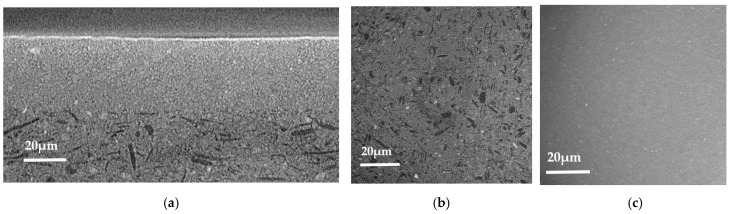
X-ray tomography images of the raw sample: (a) cross-section along the *X*-axis; (b) cross-section of the anode along the *Y*-axis; (c) cross-section of the AFL along the *Y*-axis.
